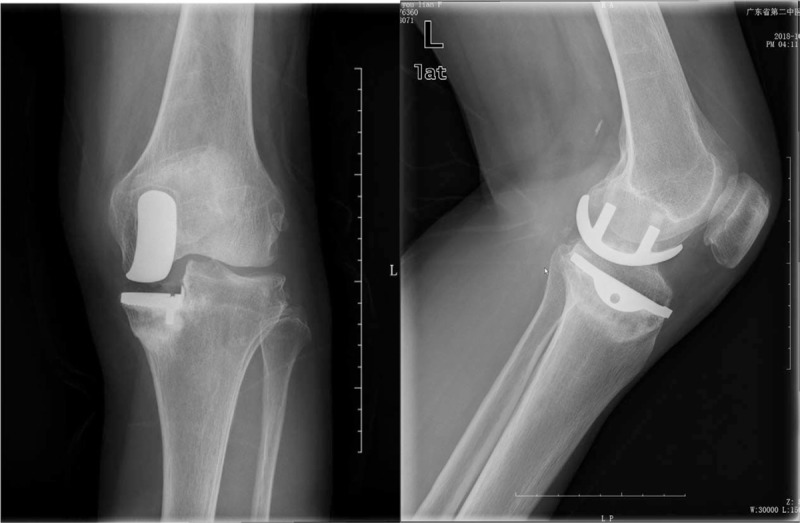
Radiograph after 1 year showing the fracture lines are better.
